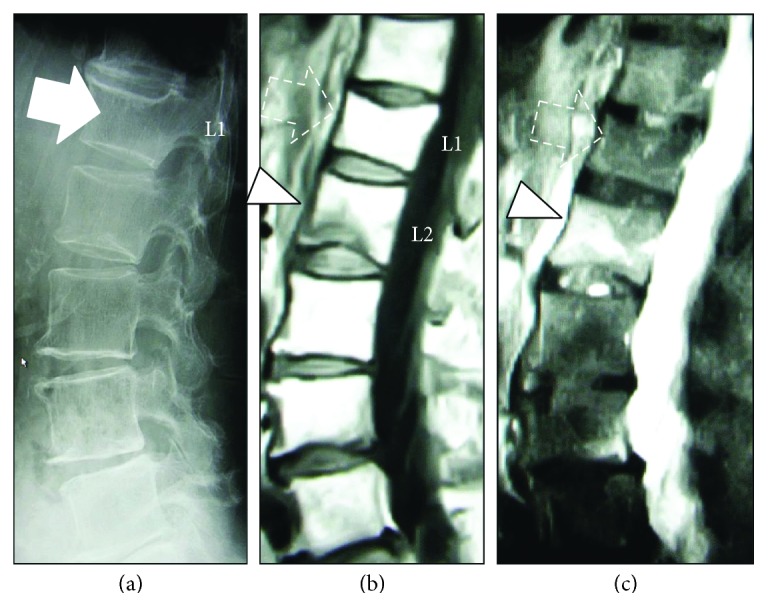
A 71-year-old woman with osteoporosis (*T*-score <−2.5 SD) and a history of no falls/injuries. (a) Plain lumbar radiography showed a possible L1 fracture (solid arrow). (b) T1-weighted MRI showed a compression fracture at the caudal adjacent L2 vertebral body (arrowheads) with a high intensity change in the T2-STIR image (c) not at the presumed level (dotted arrow) (c).
